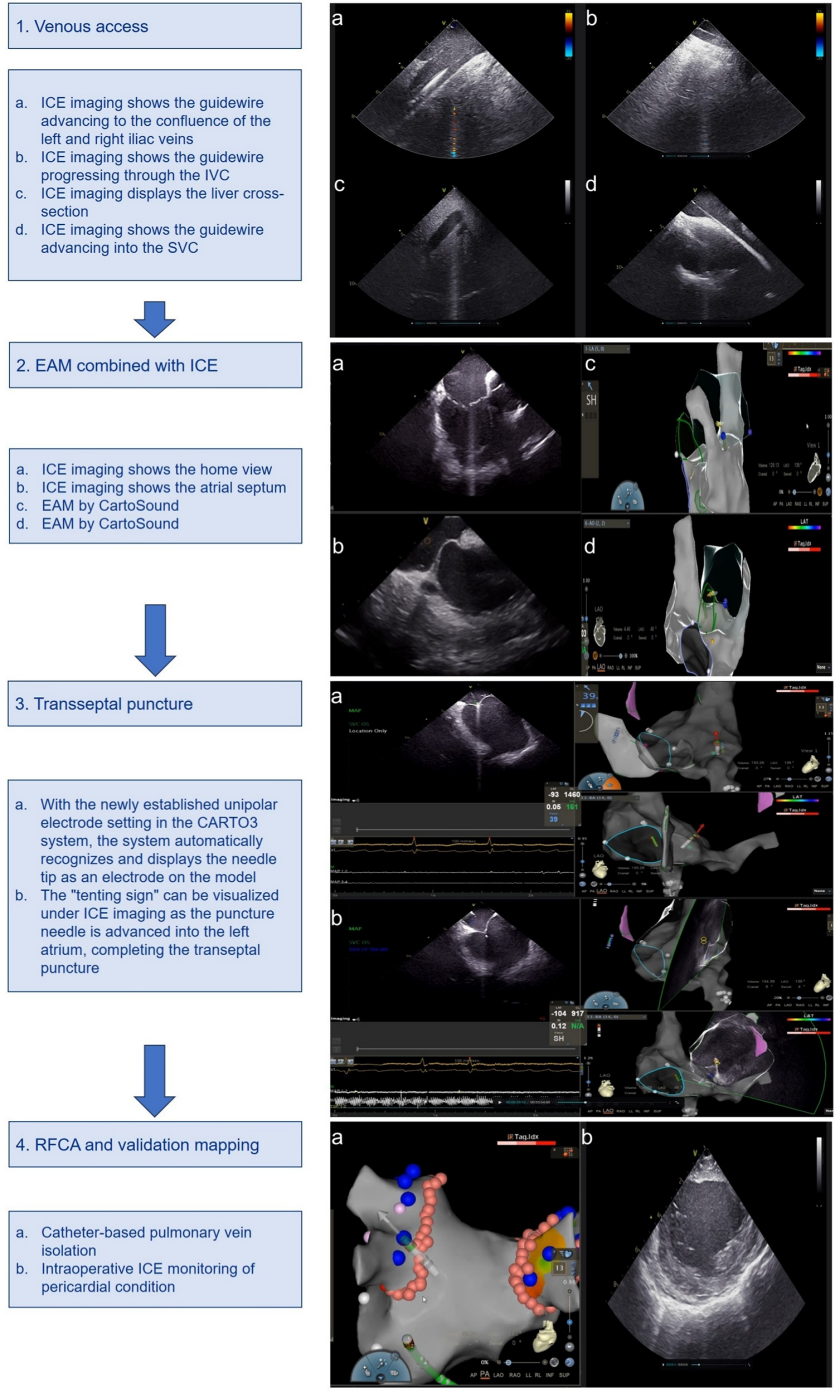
Zero-fluoroscopy workflow using ICE combined with EAM. ICE, intracardiac ultrasound; EAM, electroanatomic mapping; IVC, inferior vena cava; SVC, superior vena cava.
